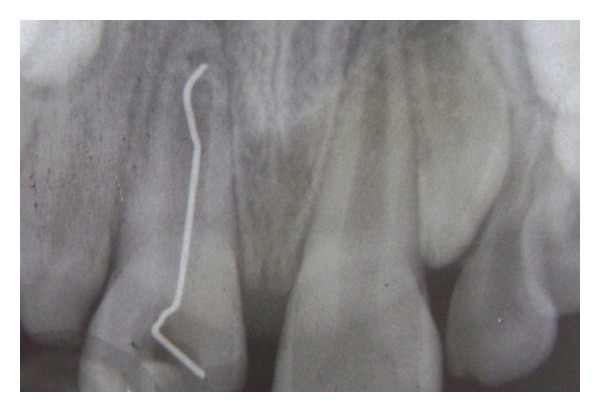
Intraoral periapical radiograph showing stapler pin in the pulp chamber of 11.
